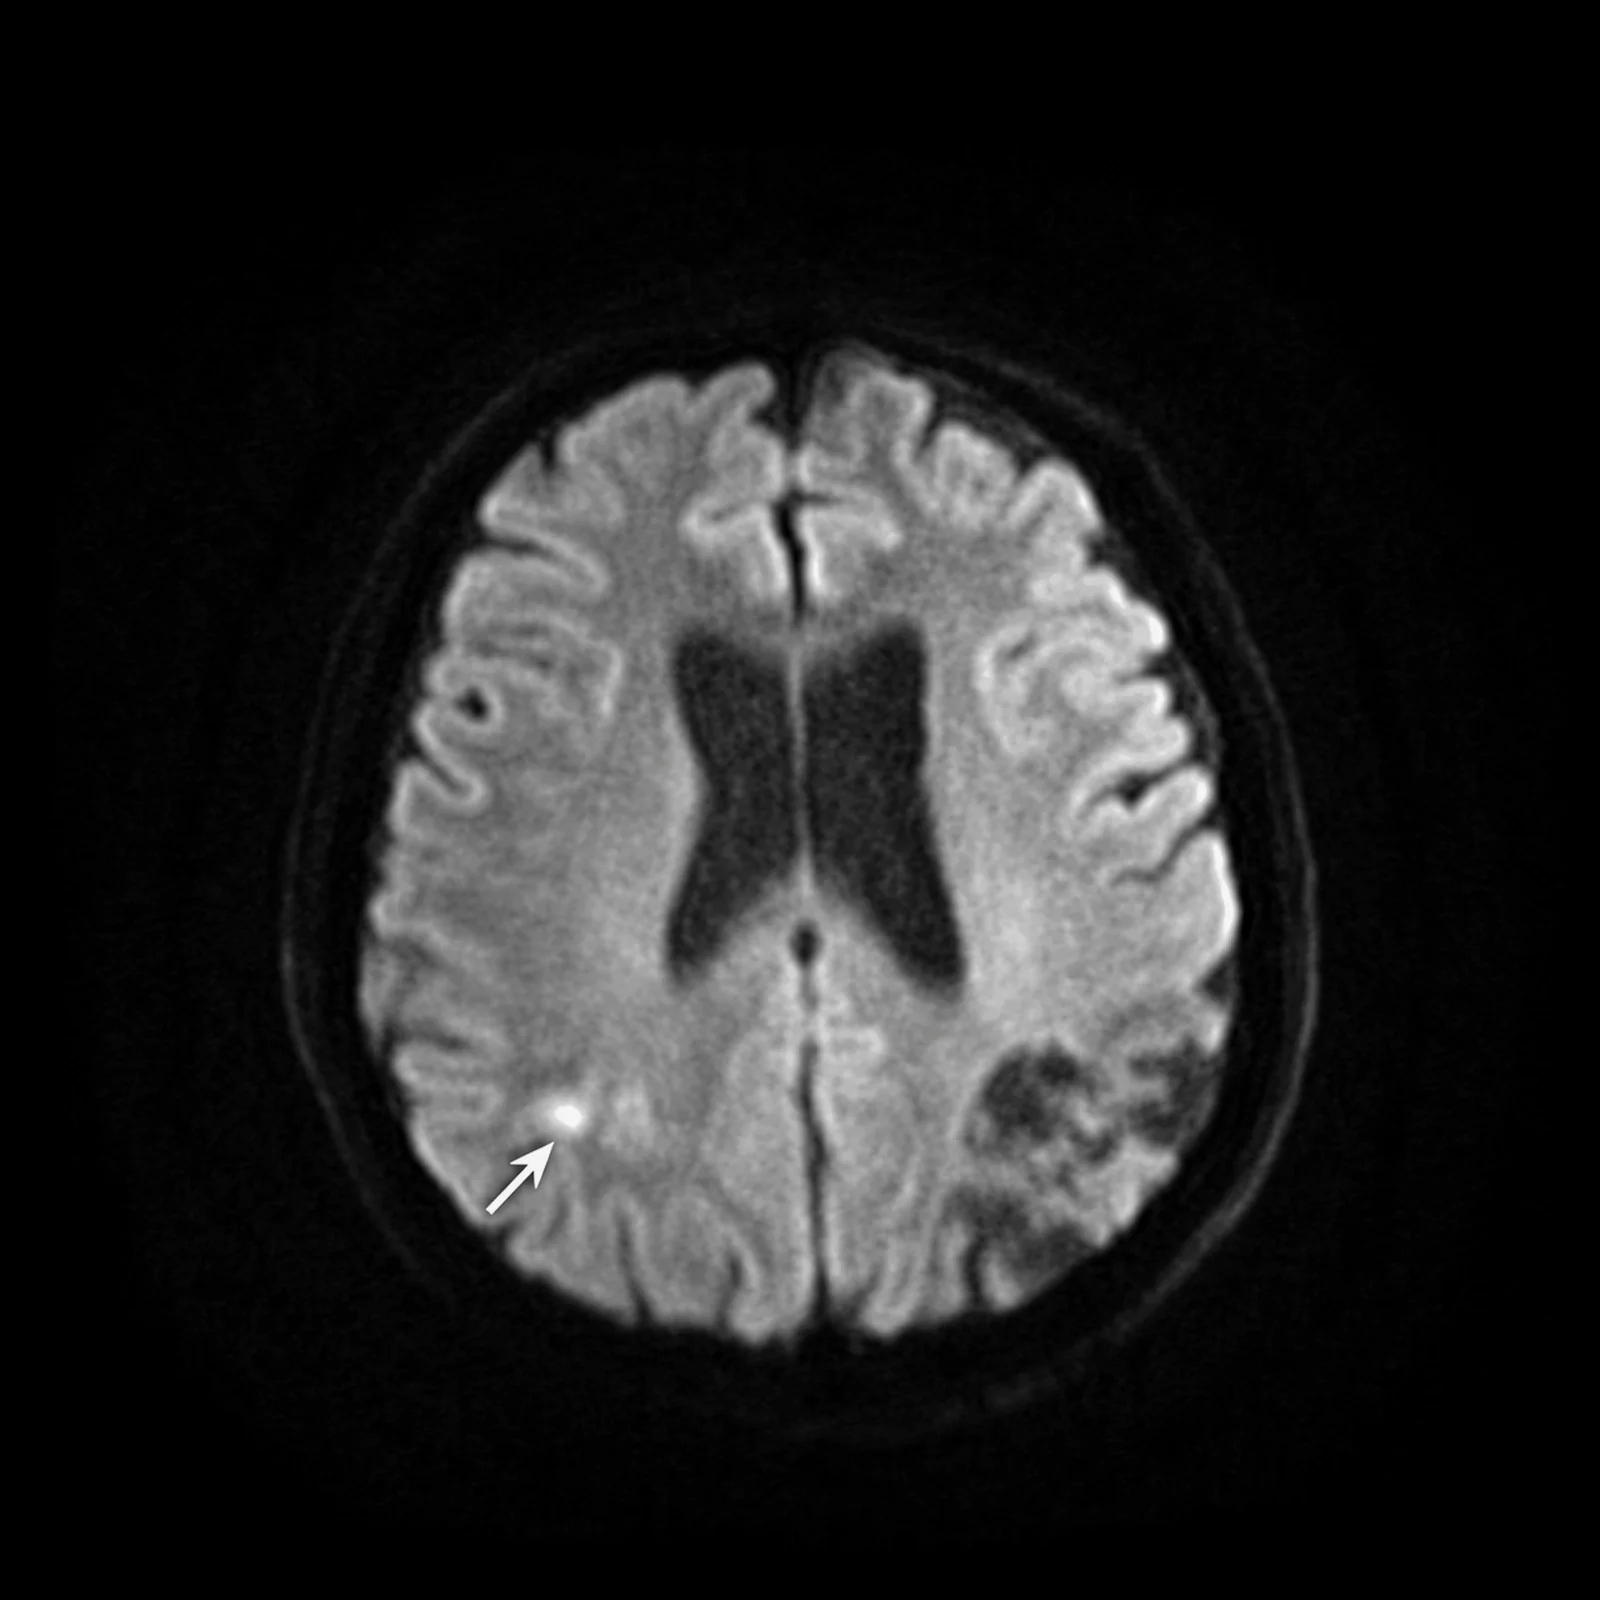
Axial diffusion-weighted MRI. Axial diffusion-weighted MRI showing a small focal hyperintense lesion in the right parietal cortico-subcortical region (arrow), consistent with an acute ischemic infarct.
